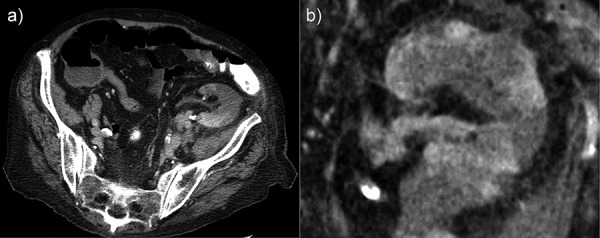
Computed tomography of patient’s abdomen. Axial (a) and coronary (b) computed tomography with contrast media showing several large infarct areas in the transplanted kidney (left iliac fossa). The renal artery is perfused. Slide size of computed tomography is 1.25 mm.
